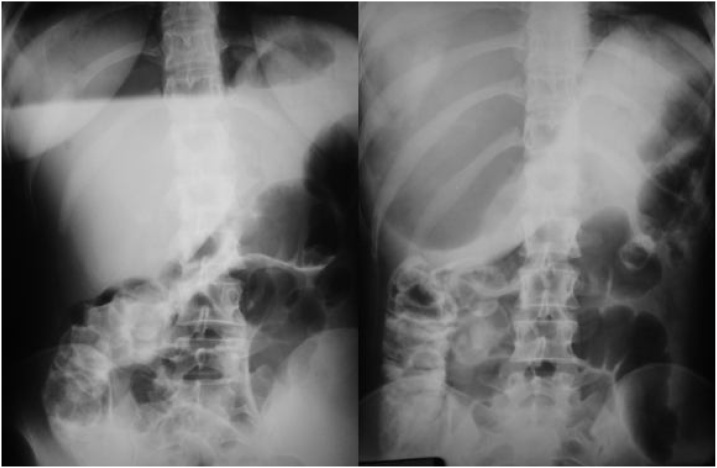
Abdominal x-ray showing gastric distension.
